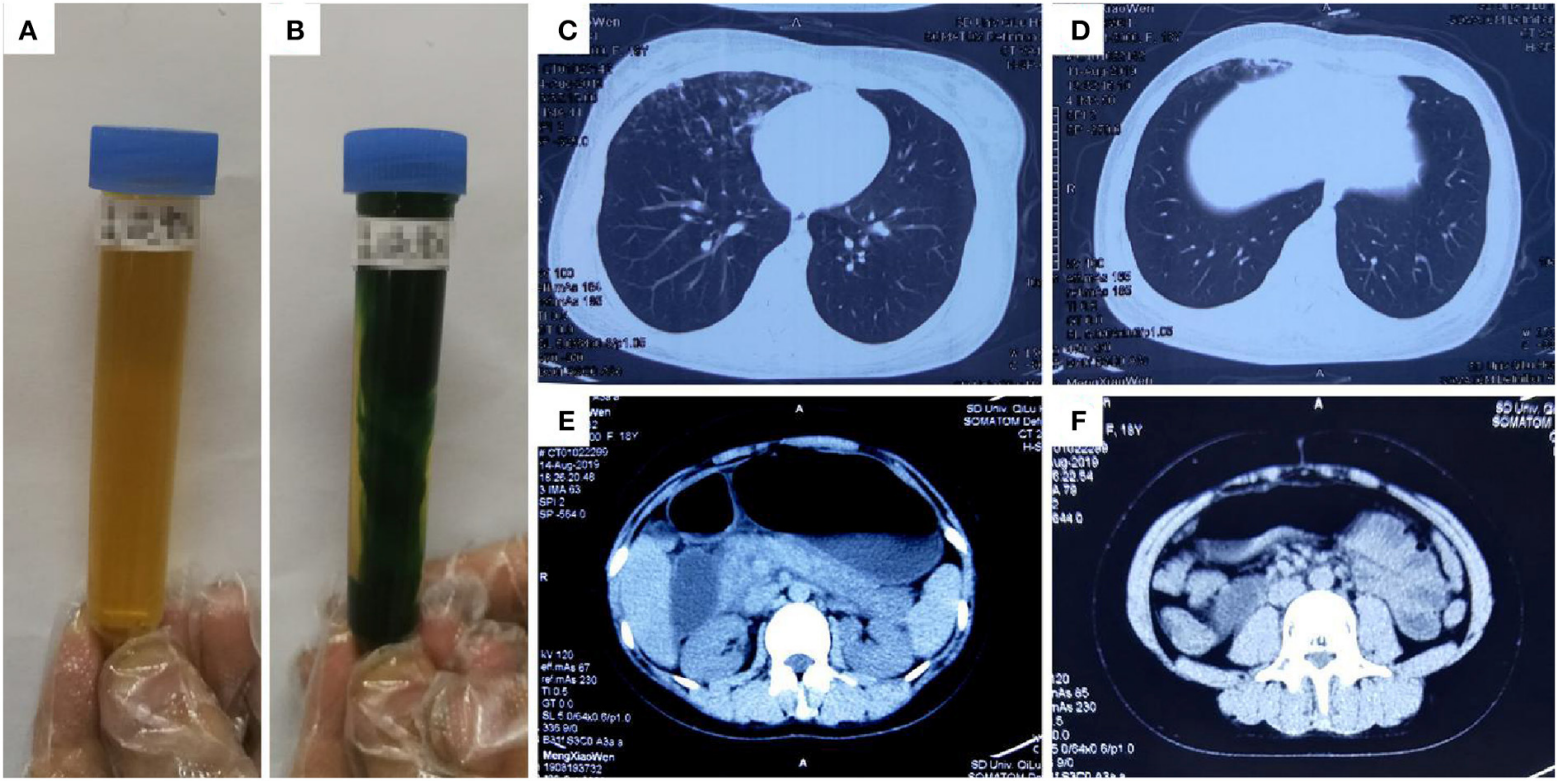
Images of urine and computerized tomography (CT) for the 18-year-old woman who ingested ~200 mL of diquat. As shown in (A), the patient's original urine was dark brown in color: after exposure to sodium dithionite, the patient's urine turned dark green (B). The images were taken 11 h after ingestion; (C,D) show lung damage, and (E,F) show gastrointestinal gas, dilatation, and fluid level; The images were taken 11 h after ingestion.
